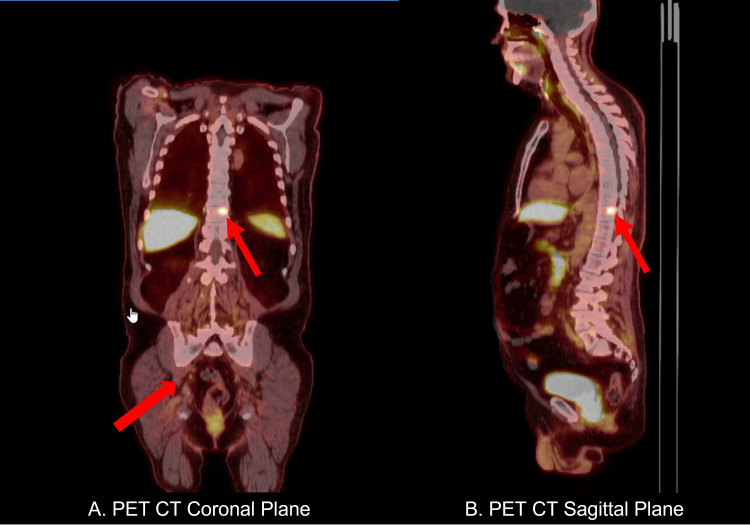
PET-CT showing metastatic osteolytic lesions in the left anterior pubic ramus and T10 vertebrae
